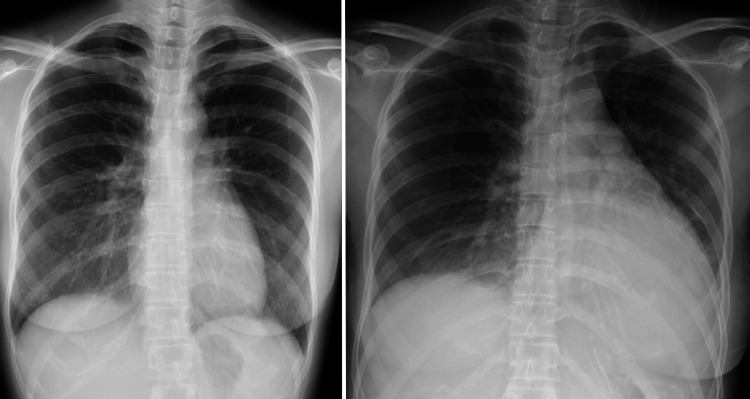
Chest X-ray Chest radiograph at the onset of DRESS (left). Chest radiograph obtained at the onset of initial heart failure, showing cardiomegaly with pulmonary congestion (right). DRESS: Drug reaction with eosinophilia and systemic symptoms.
